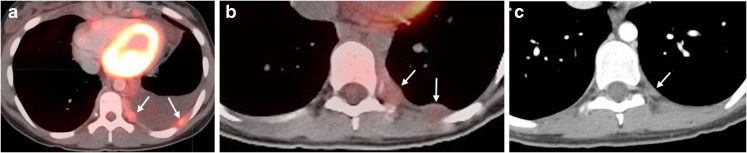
Example of complex effusion. a Pre-study PET/CT exam in a 14-year-old girl demonstrates FDG PET avidity in pleural nodules with associated moderate effusion. b, c Imaging in a 16-year-old girl with two nodules seen initially on an axial PET/CT image (b), with one small residual post-therapy nodule as seen on an axial CT image (c).
